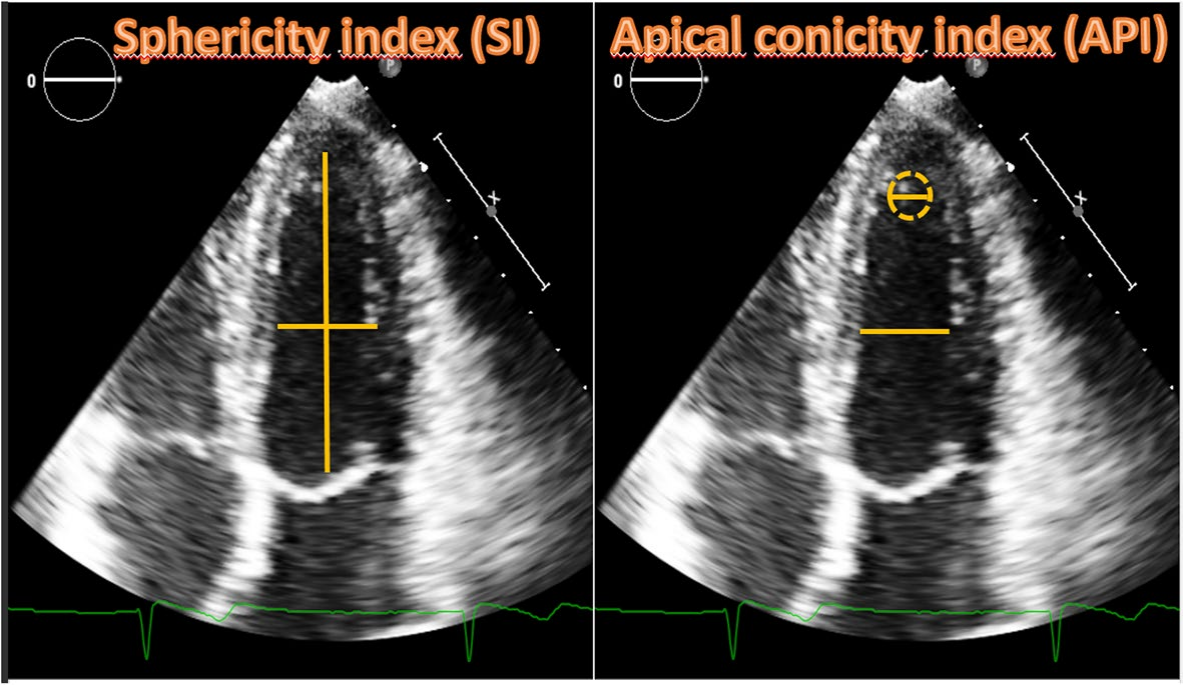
apical four-chamber view for calculation of Sphericity and Apical conicity indexes
